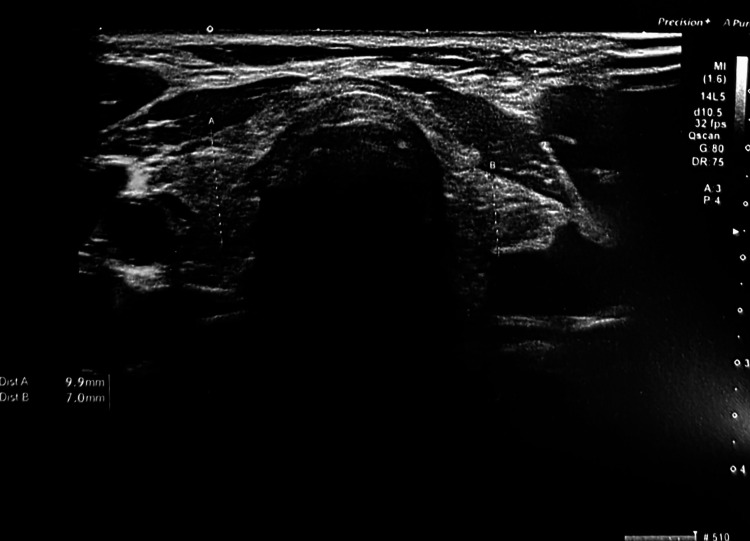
Thyroid ultrasound (anteroposterior view) The thyroid gland is hypotrophic, slightly hyperechoic, and with heterogeneous echotexture.
